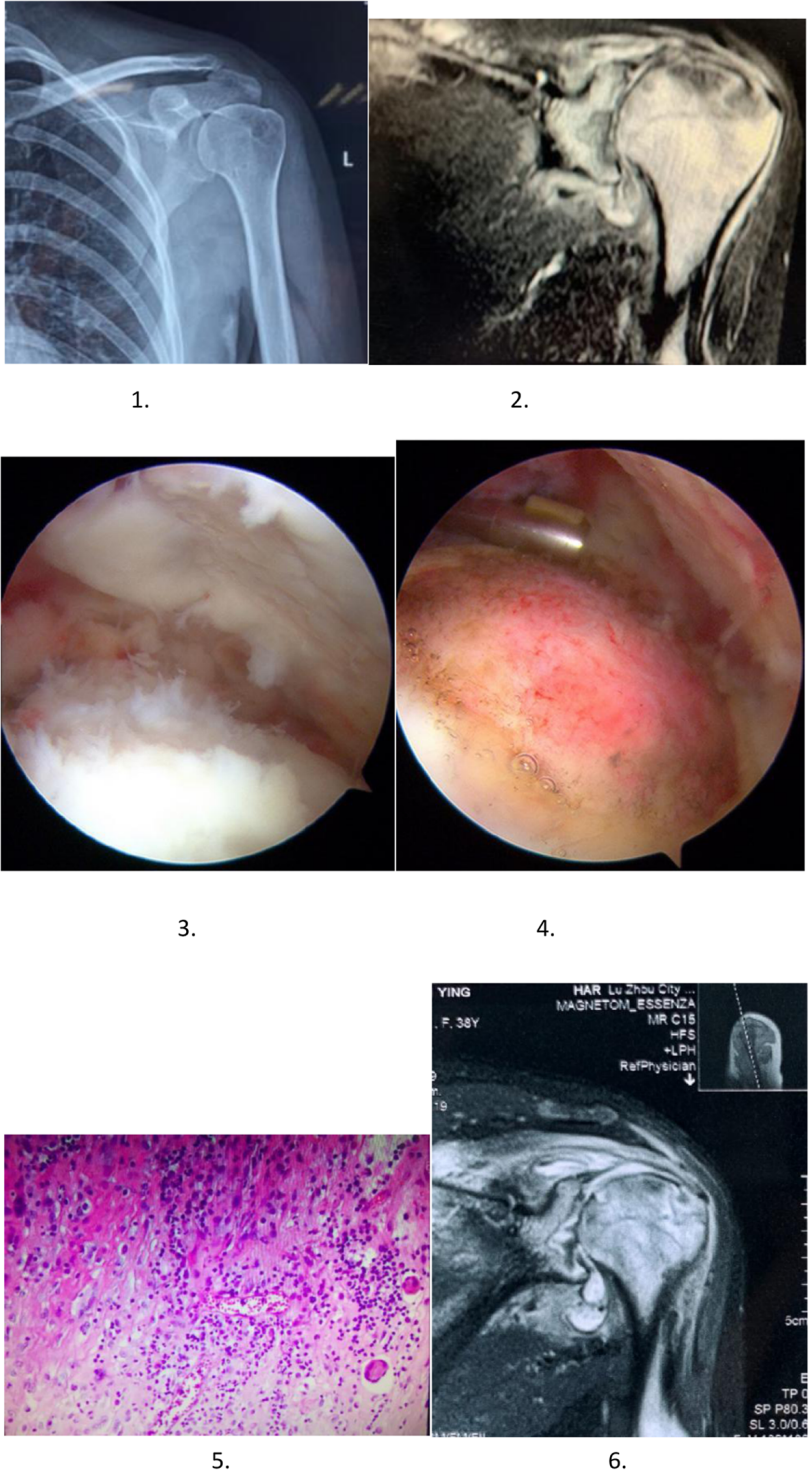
A 38-year-old female patient with joint tuberculosis of left shoulder. 1 Preoperative anteroposterior X-ray. 2 Preoperative coronal MRI. 3, 4 Arthroscopic images. 5 Postoperative pathological sections. l, 6 Coronal MRI 2 months after surgery
